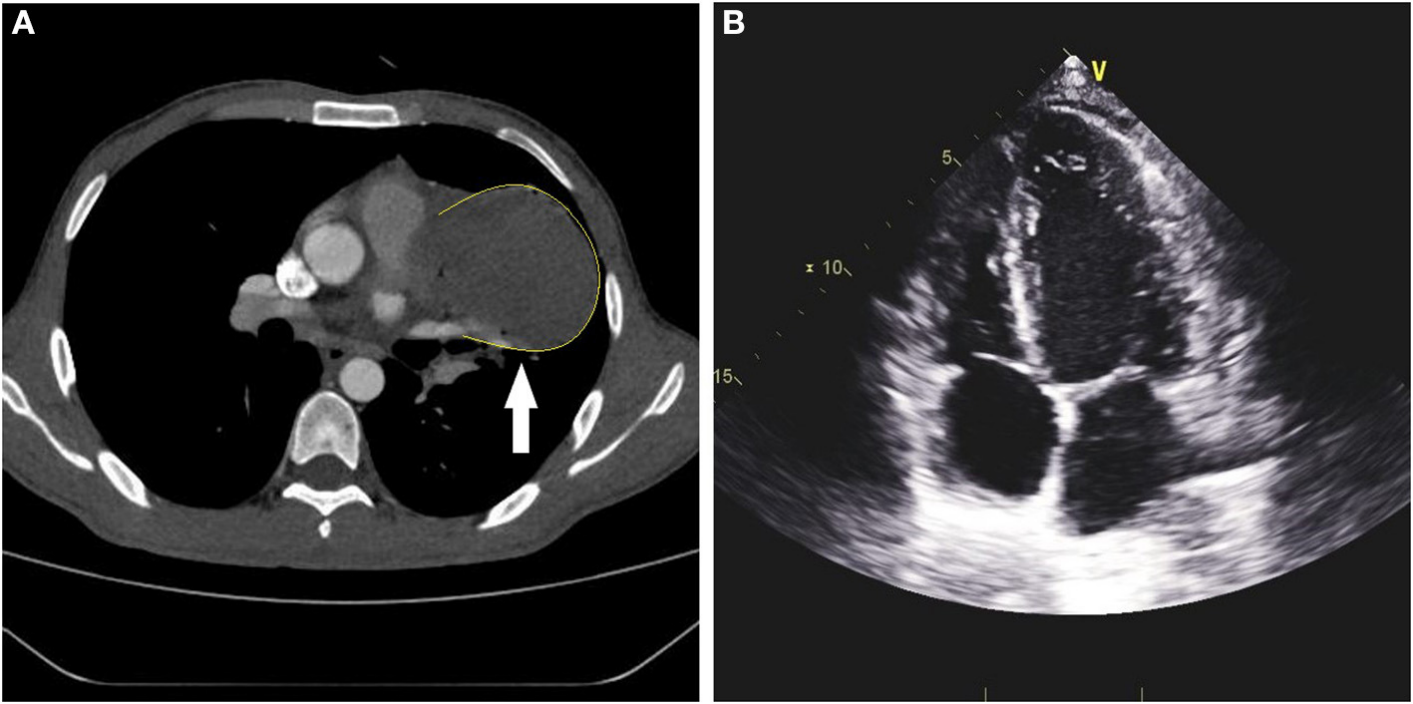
Preoperative chest CT and cardiac ultrasound. (A) Enhanced CT showed a 7.6-cm × 6.0-cm mixed-density mass shadow in the left parasternal pulmonary artery trunk of the middle mediastinum. (B) Cardiac ultrasound did not show pericardial effusion or structural abnormalities of the heart.
